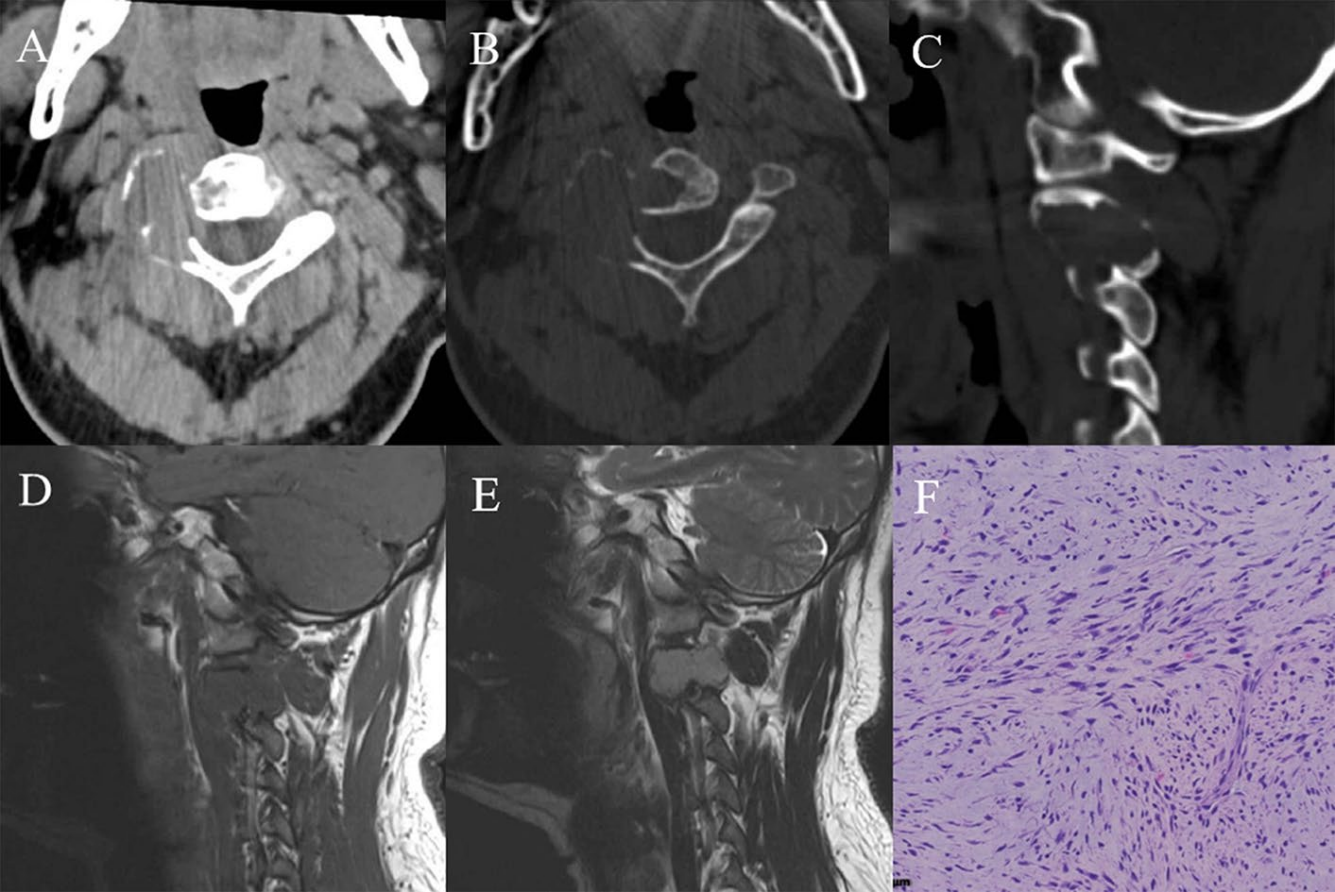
A 37-year-old male with C2 primary leiomyosarcoma. A Axial computed tomography (CT) showed an ill-defined isodense mass in the vertebral body and posterior elements of C2; B Axial CT bone window showed expansive bone destruction; C Sagittal CT bone window showed bone destruction in the vertebral body and posterior elements of C2; D T1 weighted imaging (T1WI) in sagittal view showed an isointense mass; E T2 weighted imaging (T2WI) in sagittal view showed an isointense mass; F The spindle cell in the tumor differentiated into densely packed smooth muscle cells; no tumor necrosis was observed (HE, ×400)
